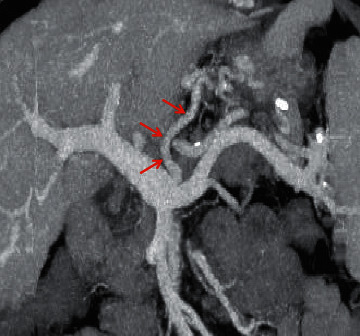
Left gastric vein. A 76-year-old female with a three-year history of autoimmune-related liver cirrhosis presented with recurrent hematemesis and melena. The coronal contrast-enhanced CT scan on the portal vein phase demonstrated the enlarged and tortuous left gastric vein (red arrow) at the lesser curvature of the stomach and the posterior wall of the left hepatic lobe.
